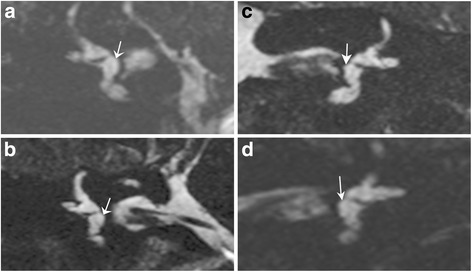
3T MRI. Four different patients suffering from Meniere disease: a, b, c and d. Coronal T2-weighted gradient-echo FIESTA-C images through the anterior and external ampullas, showing four dilated saccules with an increased saccular height > 1.6 mm (white arrows)
